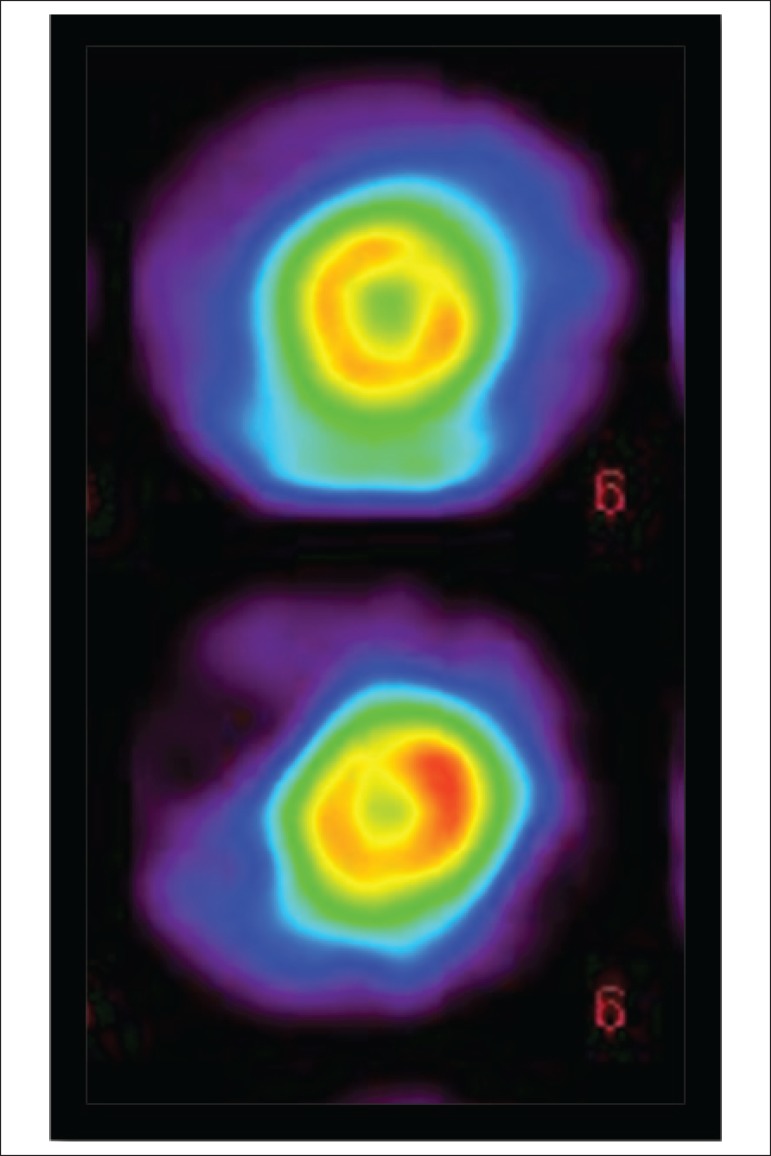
Myocardial scintigraphy with 2-methoxy isobutyl isonitrile -99mTc
